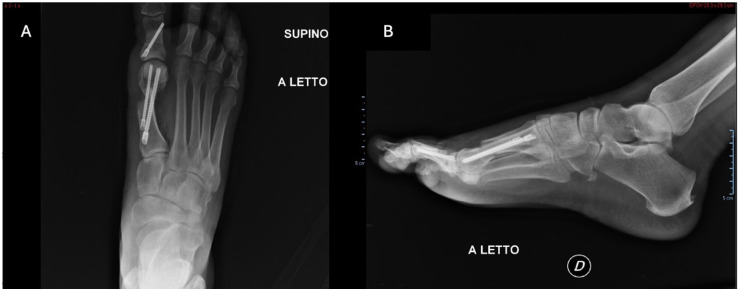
Postoperative radiographs obtained 1 month after MICA procedure: (A) anteroposterior (AP) view and (B) lateral view. MICA, minimally invasive chevron Akin.
